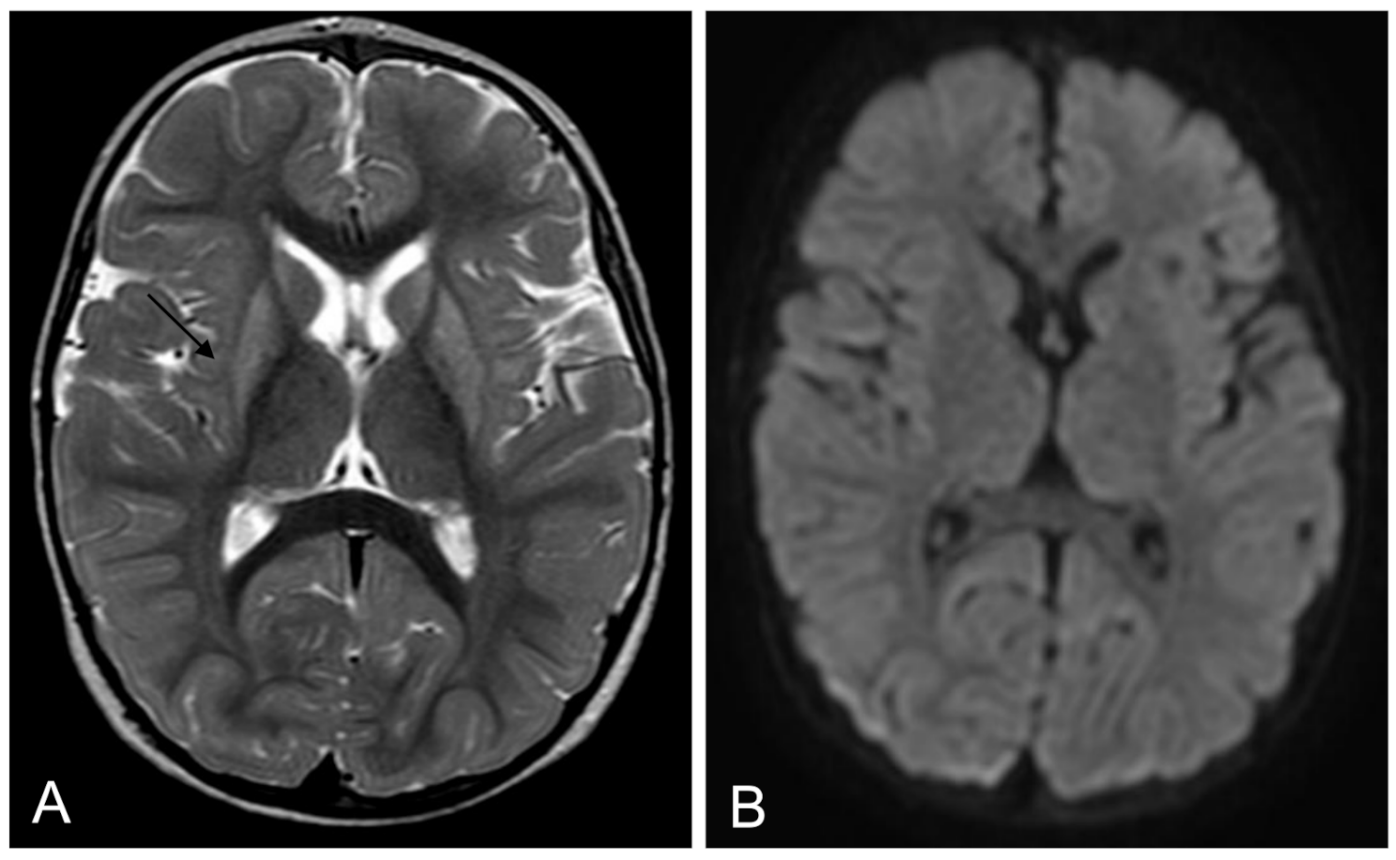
Brain MRI after 5 months. Axial T2-weighted (A) and diffusion-weighted (B) images show atrophic evolution of basal ganglia lesions and resolution of the cytotoxic edema (arrows)
